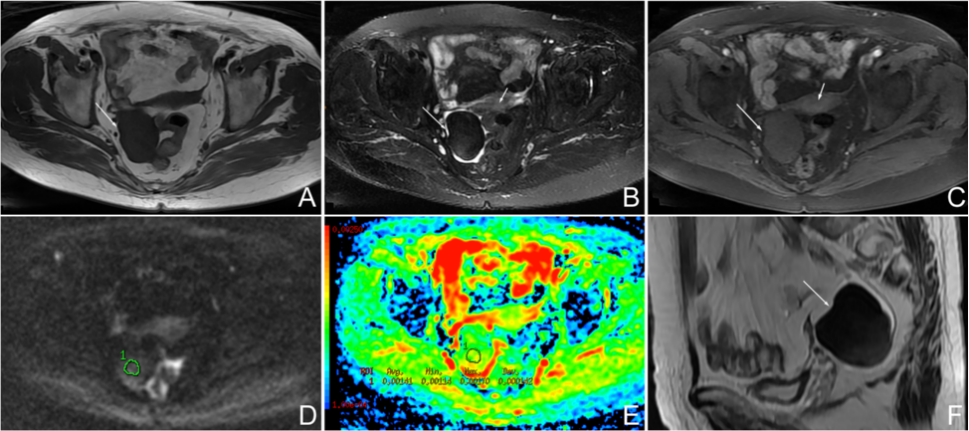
Female, 77 years, fibrothecoma smaller than 6 cm on the right ovary. a Axial T1WI showed a solid mass with homogenous isointensity (*arrow*). b On axial fat saturated T2WI, the mass showed homogenous hypointensity (*long arrow*) compared with myometrium (*short arrow*). There was no degenerative change. c On axial contrast-enhanced fat saturated T1WI, the mass (*long arrow*) revealed minor enhancement, weaker than the myometrium (*short arrow*). d On DWI, the mass appeared as a slightly high signal (grade 1). e On ADC map, the mean ADC value was 1.41 × 10^−3^ mm^2^/s. f On sagittal T2WI, the manifestation was similar to Fig. 1b
